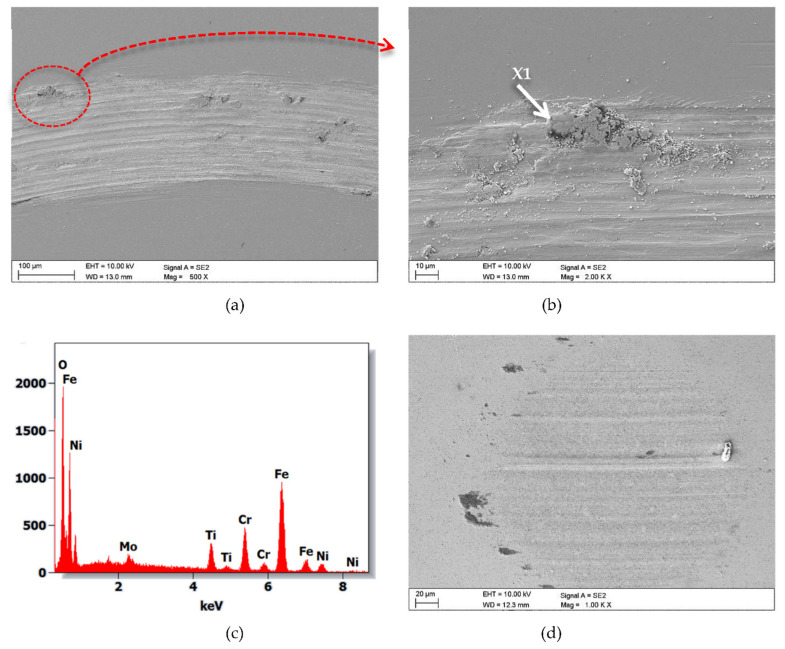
(a,b) Wear trace after the “ball-on-disc” wear test for TiO_2_/nanoTiO_2_ (Hybrid 3) bimodal coatings, (c) X-ray energy dispersive plot the area X1 as in Figure b, (d) wear place after the “ball-on-disc” wear test for cemented carbides ball as counter-sample.
